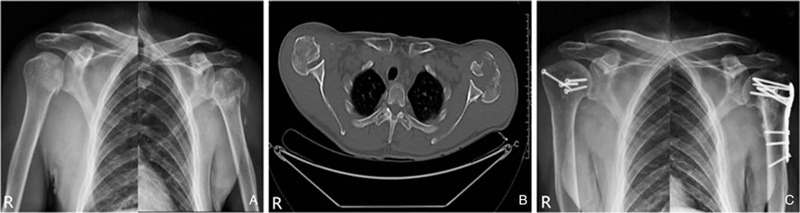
(a) Anteroposterior radiograph of the shoulders showing bilateral proximal humeral fractures; (b) axial CT of both sides confirming the dislocation and showing a posterior 4-part fracture-dislocation of the left shoulder and a posterior 2-part fracture-dislocation of the right shoulder; (c) postoperative X-ray showing good reduction of bilateral fractures and appropriate implants position.
